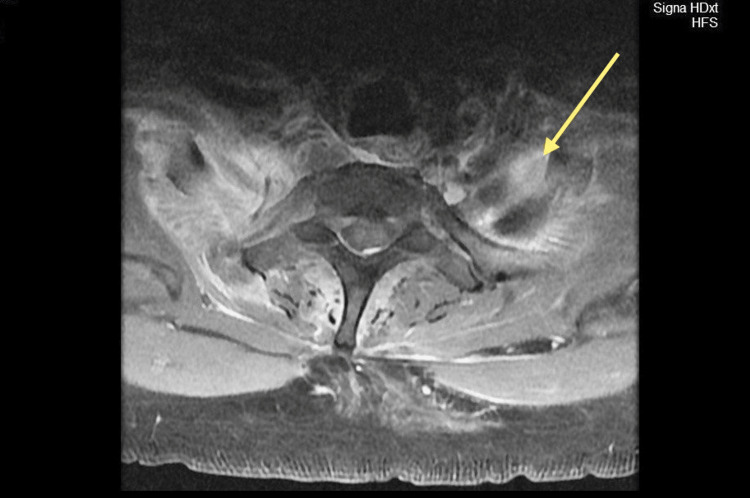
MRI of the cervical spine in transverse section showing a CEA, as indicated by the yellow arrow. CEA: Cervical epidural abscess
